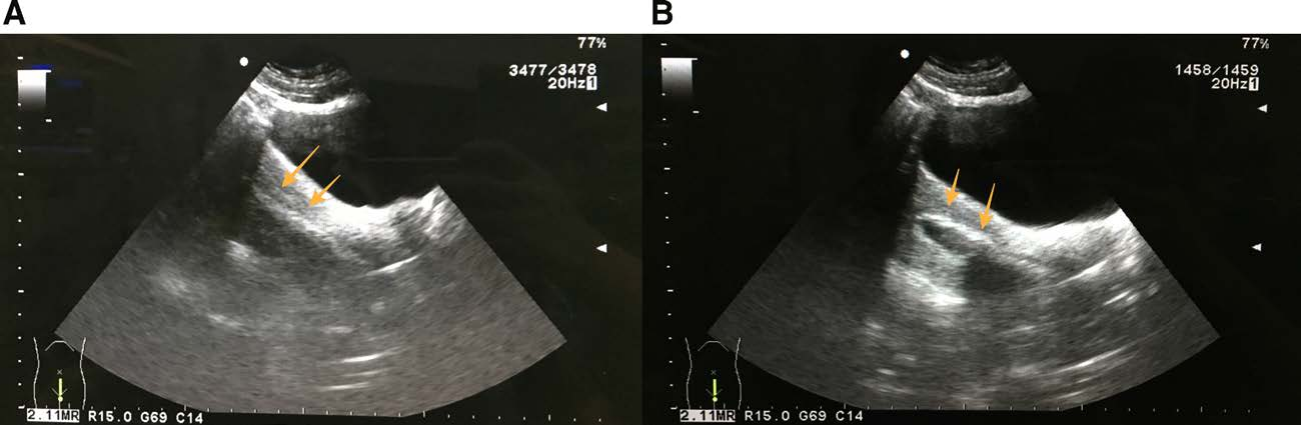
(A) US depicting modified balloon catheter with intrauterine placement. (B) US depicting catheter balloon dilation to resect adhesions. US = ultrasound.
